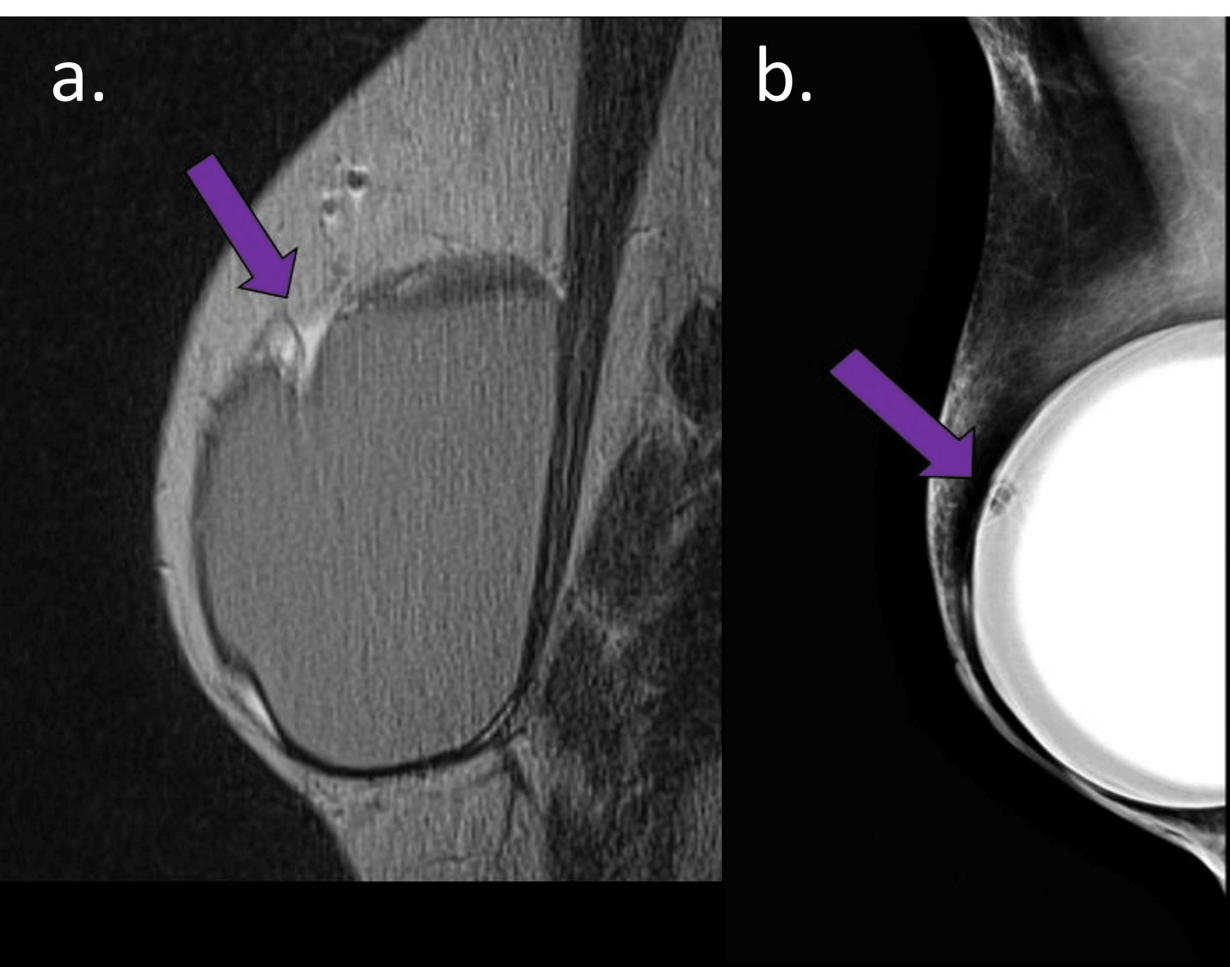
Mammography findings of acellular dermal matrix (ADM)-related complications A 60-year-old woman with a mastectomy and reconstruction with silicone implant and ADM for three years. (a) Sagittal DP-weighted sequence and (b) and breast mammography in oblique view. (a) Foldable intracapsular hyposignal mass (purple arrow) associated with intracapsular collection on MRI Mammogram: (a) In the same site as MRI findings and (b) radial fold with hypotransparency material inside (purple arrow)
